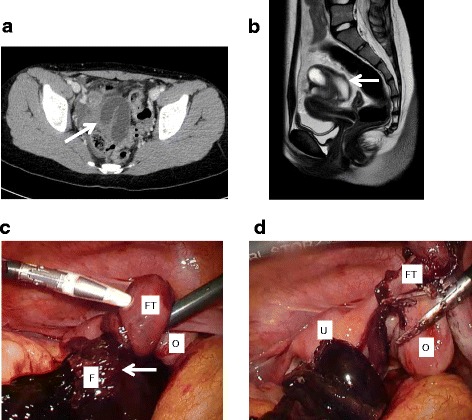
Pre-operative and peri-operative images in case 2. a Contrast-enhanced computed tomography shows a dilated fluid-filled tubular structure (*arrow*). b Sagittal T2-weighted magnetic resonance imaging shows a hyperintense tubular structure (*arrow*) positioned on the superior aspect of the uterus. c The right fallopian tube was twisted, and the fimbria was dilated, dusky in appearance, and completely necrotic (*arrow*). *F* right fimbria, *FT* right fallopian tube, *O* right ovary. d After removal of the necrotic fimbria and distal side of the fallopian tube. The uterus and the right ovary were normal. *U* uterus, *FT* right fallopian tube, *O* right ovary
